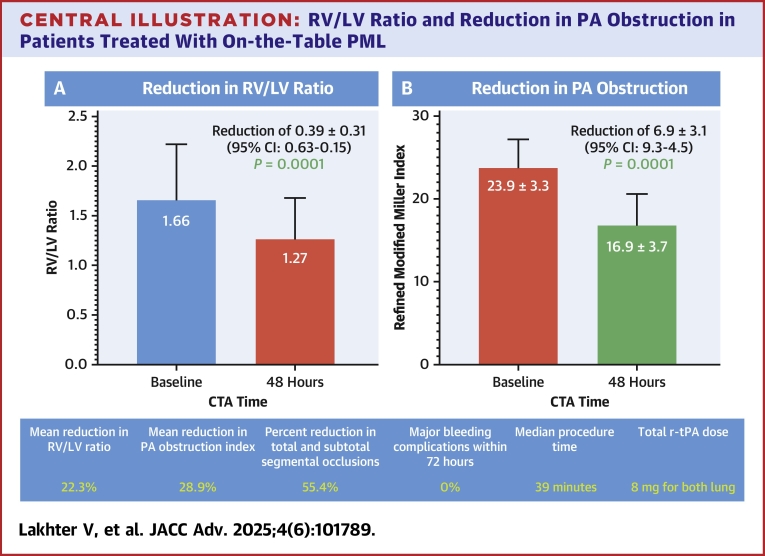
RV/LV Ratio and Reduction in PA Obstruction in Patients Treated With On-the-Table PML CTA-based RV/LV ratio at 48 hours after pharmacomechanical catheter-directed thrombolysis decreased from a baseline of 1.66 down to 1.27 (22.3% reduction) (A). Pulmonary artery obstruction based on the Refined Modified Miller Index decreased from 23.9 to 16.9 (28.9% reduction) 48 hours after treatment (B). LV = left ventricular; RV = right ventricular; other abbreviations as in Figures 1 and 2.
